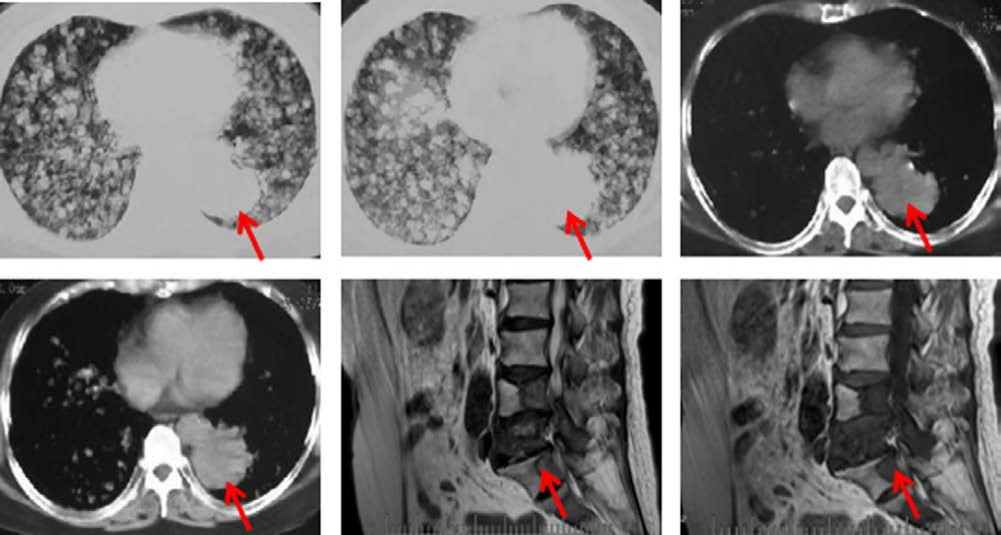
The chest CT scan demonstrated left lower lobe mass and double lung diffuse multiple metastasis, the MRI scan demonstrated bone metastasis at baseline. CT=computed tomography, MRI=magnetic resonance imaging.
